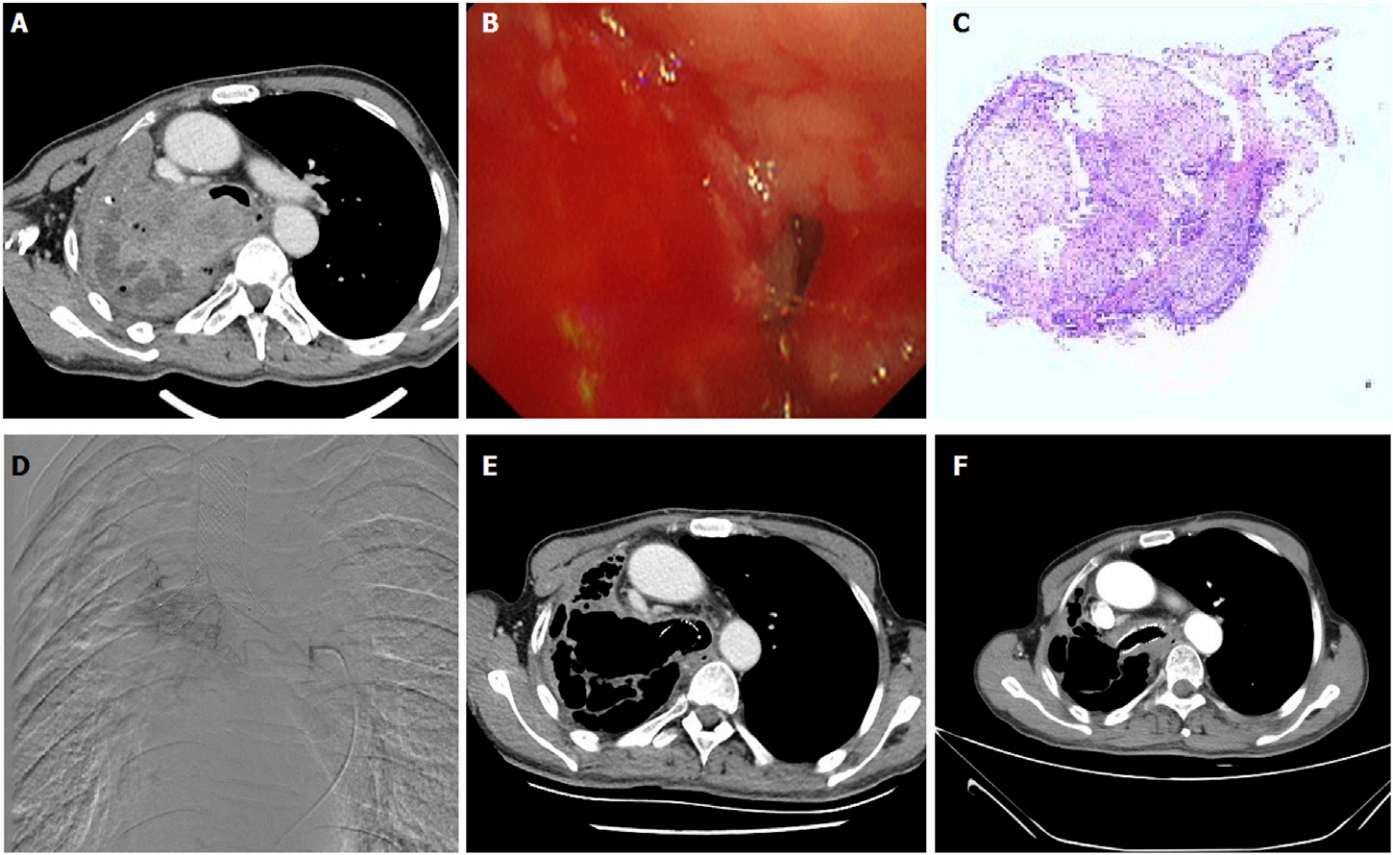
A 65-years male treated by CalliSpheres beads for advanced squamous cell carcinoma. (A) Computed tomography examination on admission revealed malignant tumor, severe bronchial stenosis and complete atelectasis of right lung. (B) Fibrobronchoscopy revealed a right main bronchial occlusion. (C) Pathological diagnosis of squamous cell carcinoma by biopsy of the lesion. (D) The right bronchial artery was the blood supply artery of the tumor, and was embolized by drug-loaded beads. (E) The right lung tumor was found to shrink after 1.5 months’ follow-up. (F) The airway stent was in good position and the tumor was almost disappeared after 1 year.
